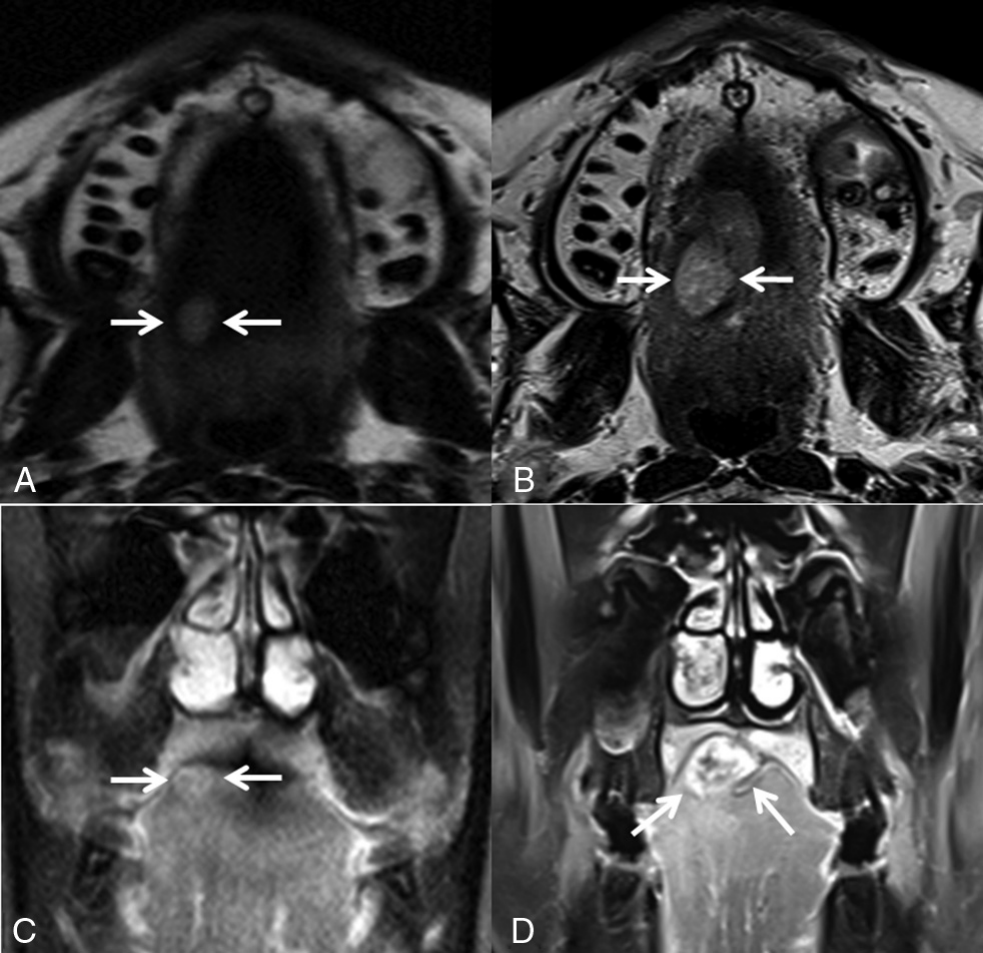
Comparison of previous and present oropharyngeal MRI: (A) the oval-shaped homogeneous mass measuring approximately 1.0 cm × 0.8 cm × 0.8 cm appeared with high signal intensity on a previous T2-weighted image, and (B) the present axial T2-weighted image showing slow-growing 17-year standing lesion with heterogeneous high signal intensity on the right side of the tongue. (C) Previous coronal contrast-enhanced T1-weighted MR image showing homogenously weak enhancement of the mass, and (D) the present MR image showing markedly heterogenous enhancement of the slow-growing mass.
